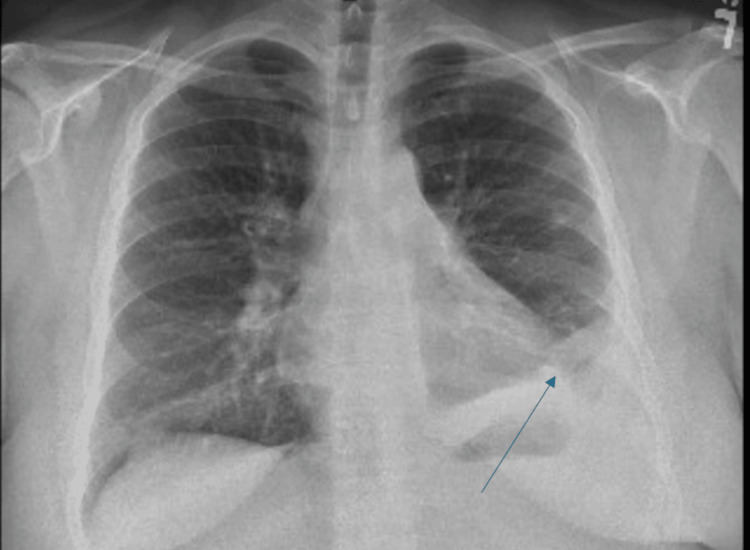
Arrow pointing to area on chest X-ray of opacification
